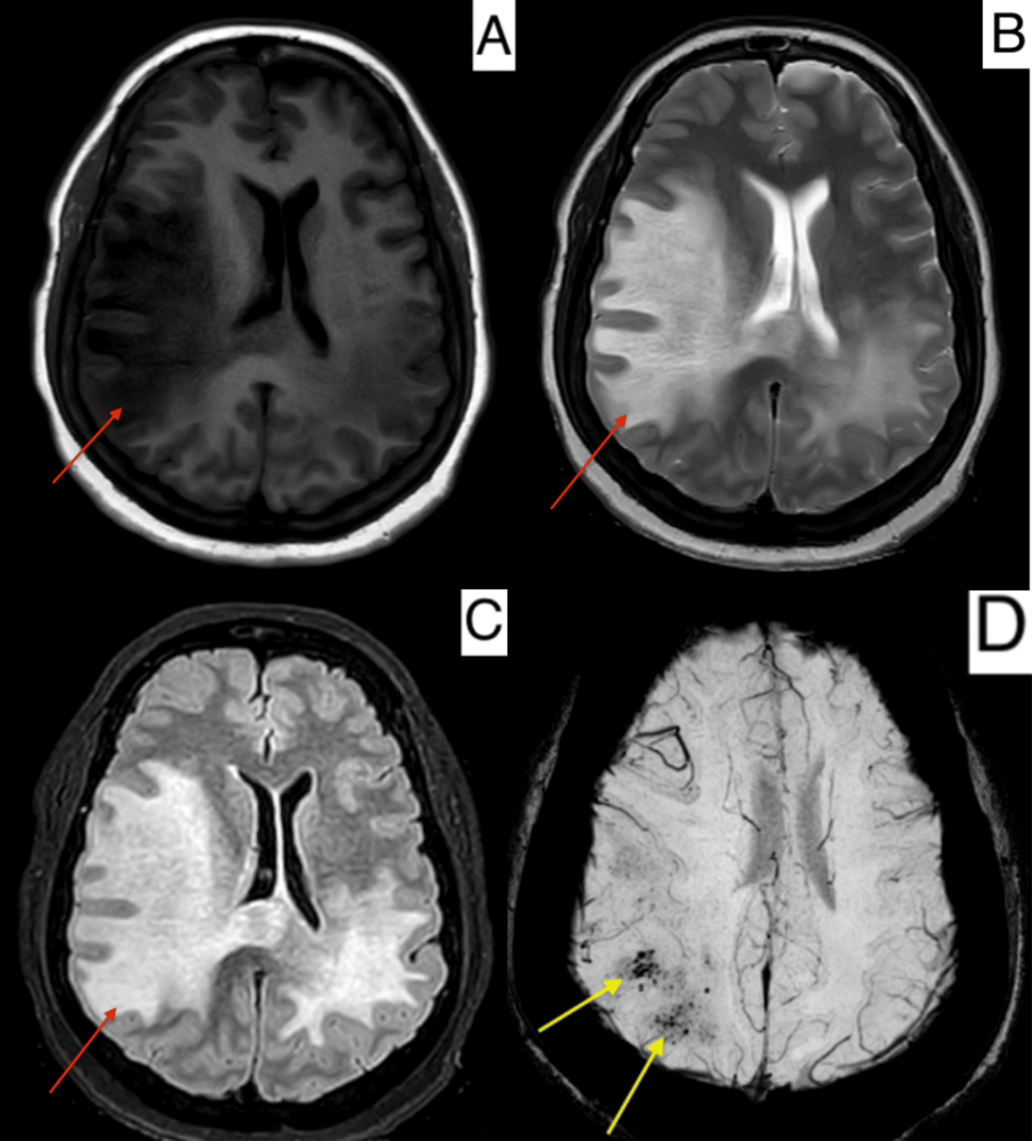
MRI of the brain. T1-weighted image (A), T2-weighted image (B), and T2-FLAIR image (C) showing significant progression of the disease along the splenium of the corpus callosum into the right fronto-parietal-temporal white matter (red arrow). SWI image (D) showing multiple hemorrhagic foci within the right parietal white matter (yellow arrows) June 10, 2024 MRI: magnetic resonance imaging, FLAIR: fluid-attenuated inversion recovery, SWI: susceptibility-weighted imaging
